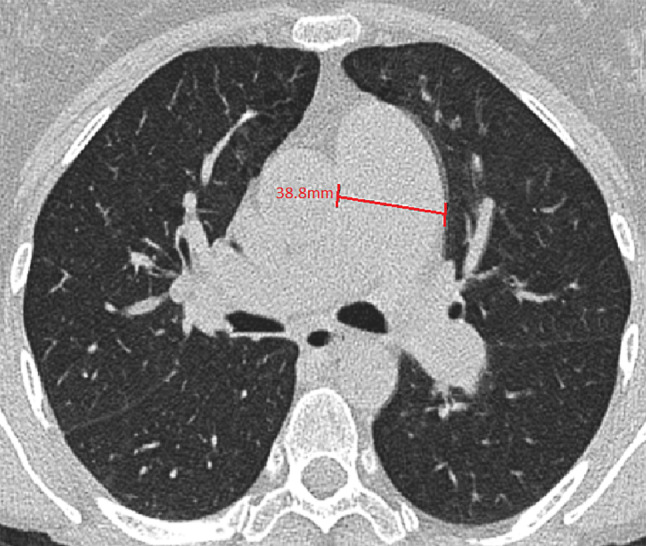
Example of a measurement of the main pulmonary artery in the transverse plane at the level of the main pulmonary artery on CT scan
